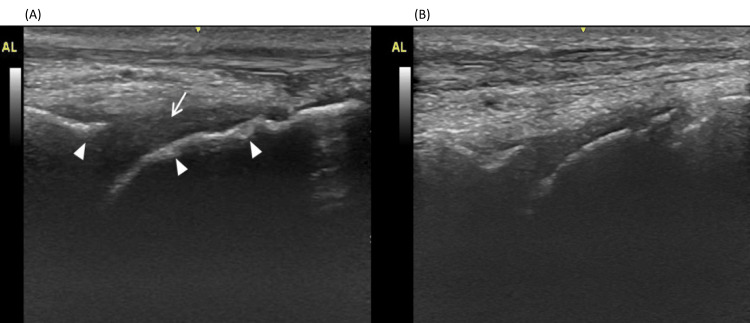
Ultrasonographic images of (A) Case 1 in September 2019 before albutrepenonacog alfa (rFIX-FP) treatment; image shows the left ankle joint, where liquid storage (arrow) and osteochondral damage (arrowheads) are observed and (B) in April 2020 while receiving rFIX-FP 3500 units (60.3 IU/kg) once weekly.
